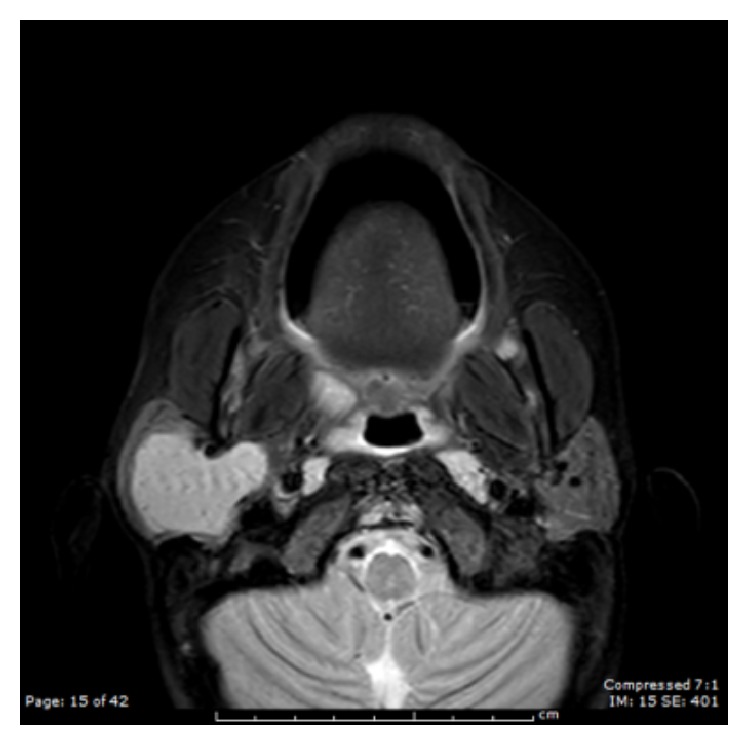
Axial T2 weight MRI shows a hyperintense lesion.
